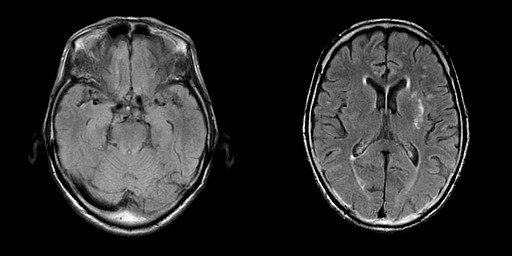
The brain MRI findings. The axial fluid-attenuated inversion recovery (FLAIR) images showed a small hyperintense lesion in the left putamen. Neither generalized nor focal cortical atrophy suggestive of Alzheimer disease or other degenerative dementias was noted.
